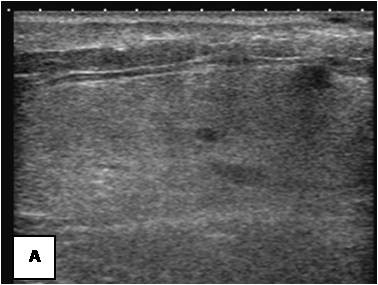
Ultrasonography exhibits a mild heterogeneous hyperechoic mass.
